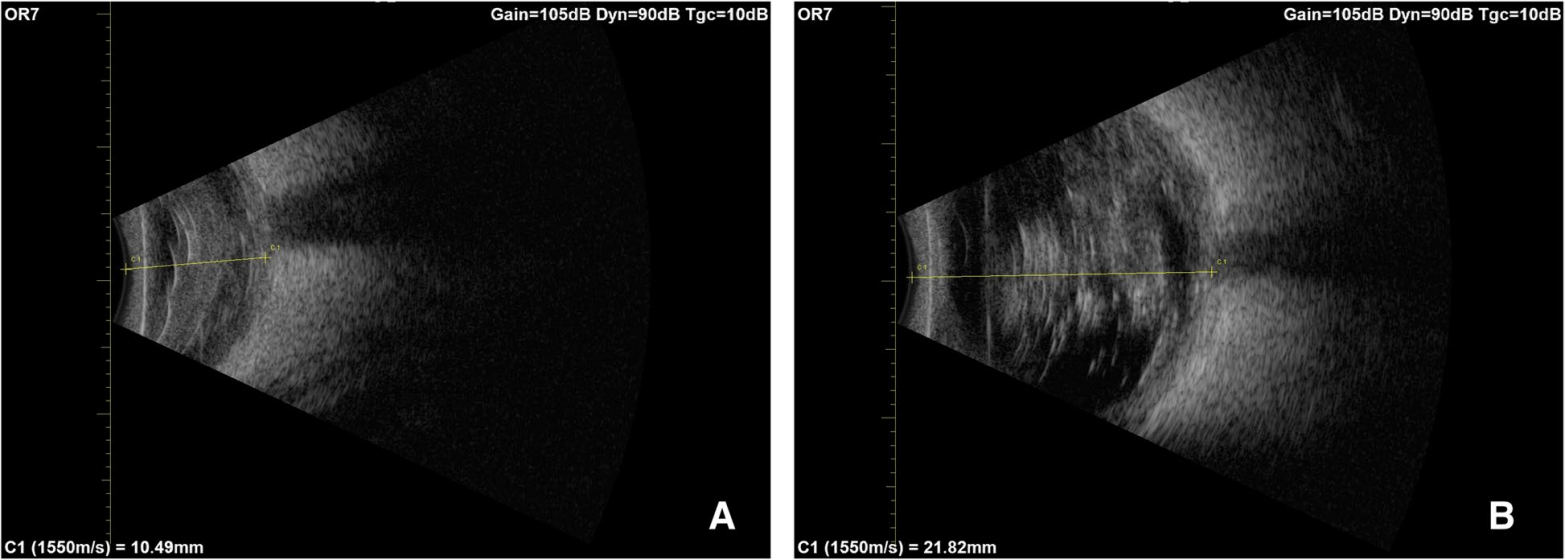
Ultrasound appraisal of a concave eye (A) - increase of diameter and sphericity after injection of 3.5 ml of Heptastarch solution (B). The posterior border of the lens, granular content of vitreous cavity, the sclera and the optic nerve head can be discerned
